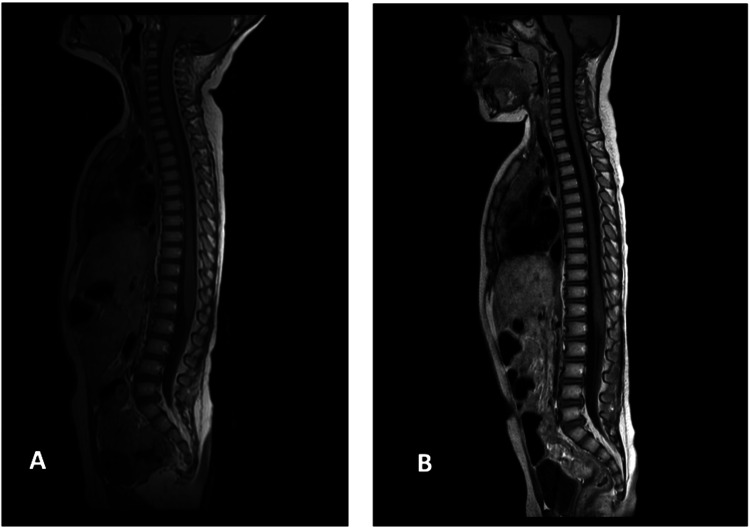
Later follow-up contrast-enhanced, sagittal T1-weighted MRI views. (A) On day 30, there is a complete radiological resolution. (B) One month post-discharge repeat MRI shows normal spinal appearance with no residual abscess or compression, confirming long-term resolution.
